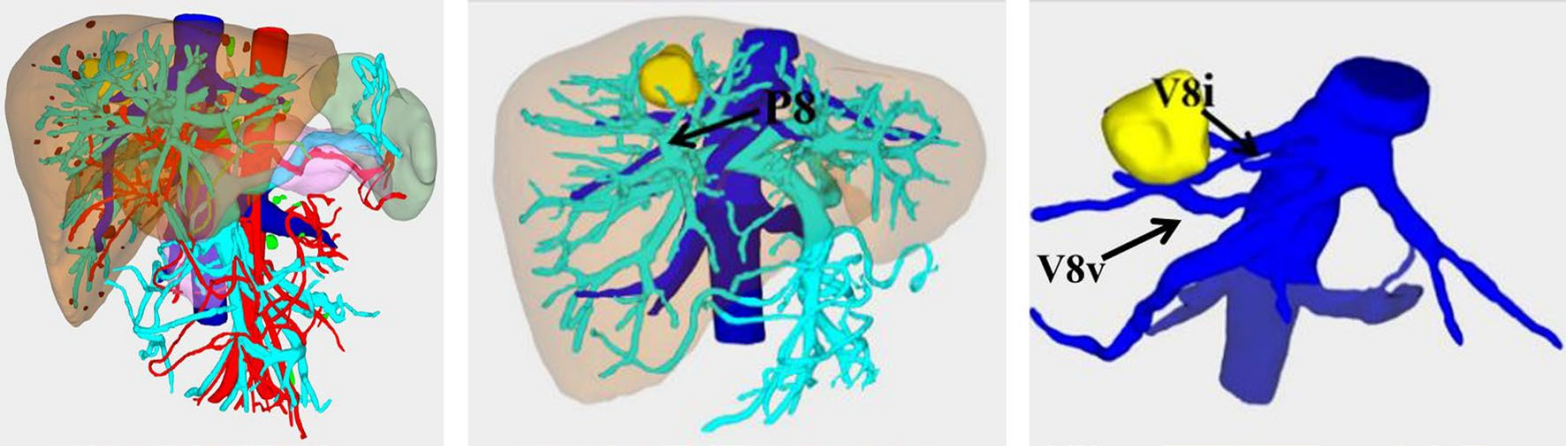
Preoperative 3D-CT reconstruction. The black arrow indicates the G8. 3D-CT reconstruction shows V8v (black arrow) joining the MHV. V8i (black arrow): tributary of MHV running between the ventral part and dorsal part of S8
